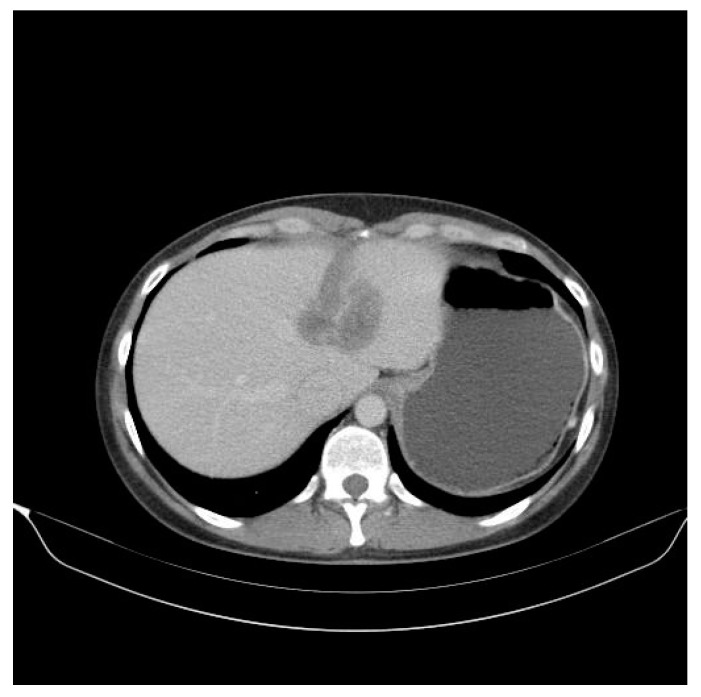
Follow-up abdominal CT performed in 2018 at 6 months after two ablation sessions of the liver metastasis with an MW of 6 and 4 min at 32 W with a temperature set at 96 degrees highlights a progressive disease of the liver metastases at 55 mm diameter.
